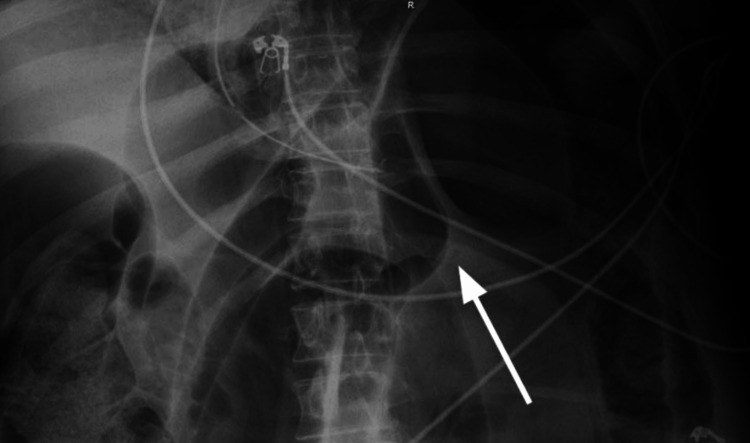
X-ray abdomen showing dilated colon (white arrow).
